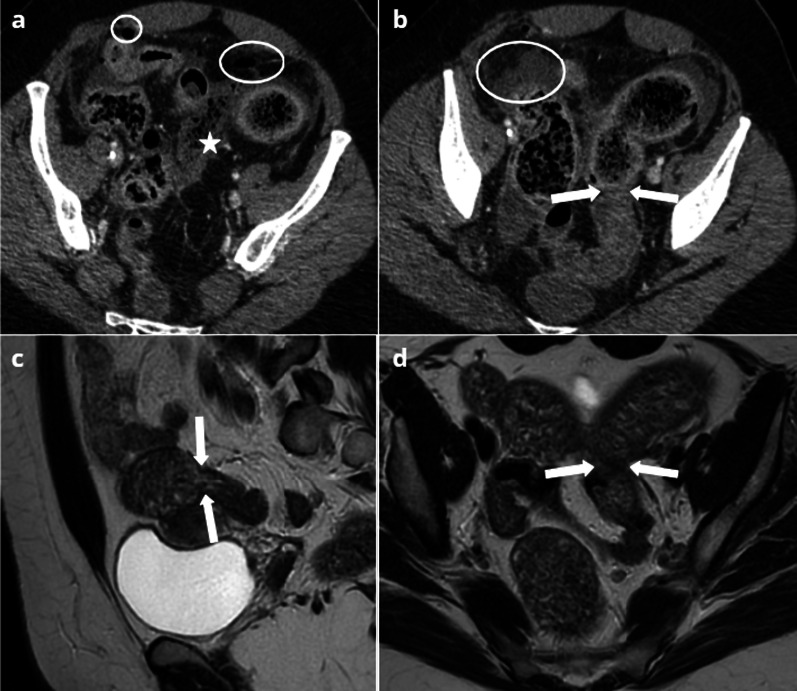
39-year-old female presenting with acute abdominal pain and abdominal tenderness at physical examination. Axial enhanced CT (a, b) shows findings of acute peritonitis: pneumoperitoneum (circles), peritoneal effusion (circles), peritoneal thickening, and fecal material within the peritoneal cavity (star). The presence of fecal material close to the sigmoid colon is suggestive of the site of perforation (a). A stenosis of the sigmoid is suspected (arrows, (b)). The patient had a history of shaving surgery for rectosigmoid endometriosis and had a pelvic MRI a few weeks before. T2W MRI (c, d) confirms the presence of a fibrotic stenosis of the sigmoid (arrows), most probably secondary to shaving surgery
